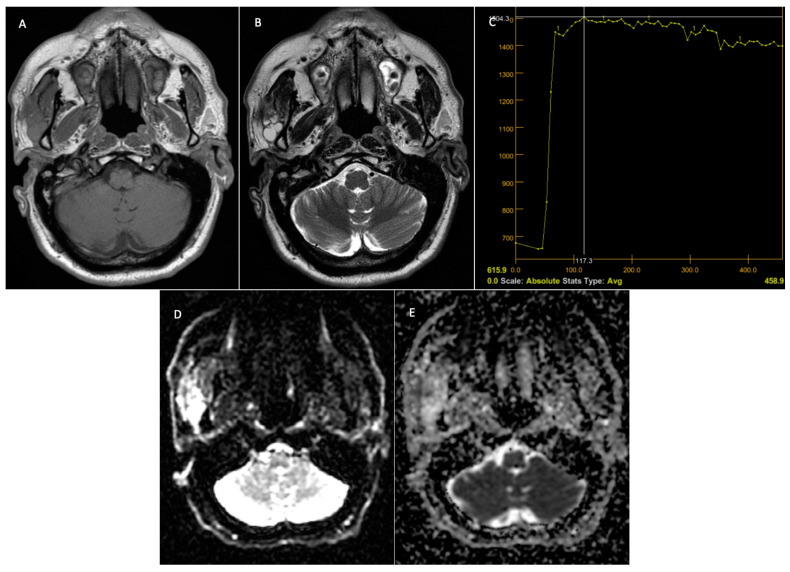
Malignant tumor—right parotid gland. Preoperative MRI. T1 sequence with a hypointense signal in the tumor (A). T2 sequence—inhomogeneous signal at the level of the lesion and the presence of micro-calcifications at the level of the lesion (B). DCE—MRI—TIC curve type B, with progressive contrast uptake followed by a plateau phase and washout (C). The DWI—MRI sequences (D) demonstrate restriction at the level of the solid component of the tumor, with the ADC map (E) having a value of 1 × 10^−3^ mm^2^/s.
